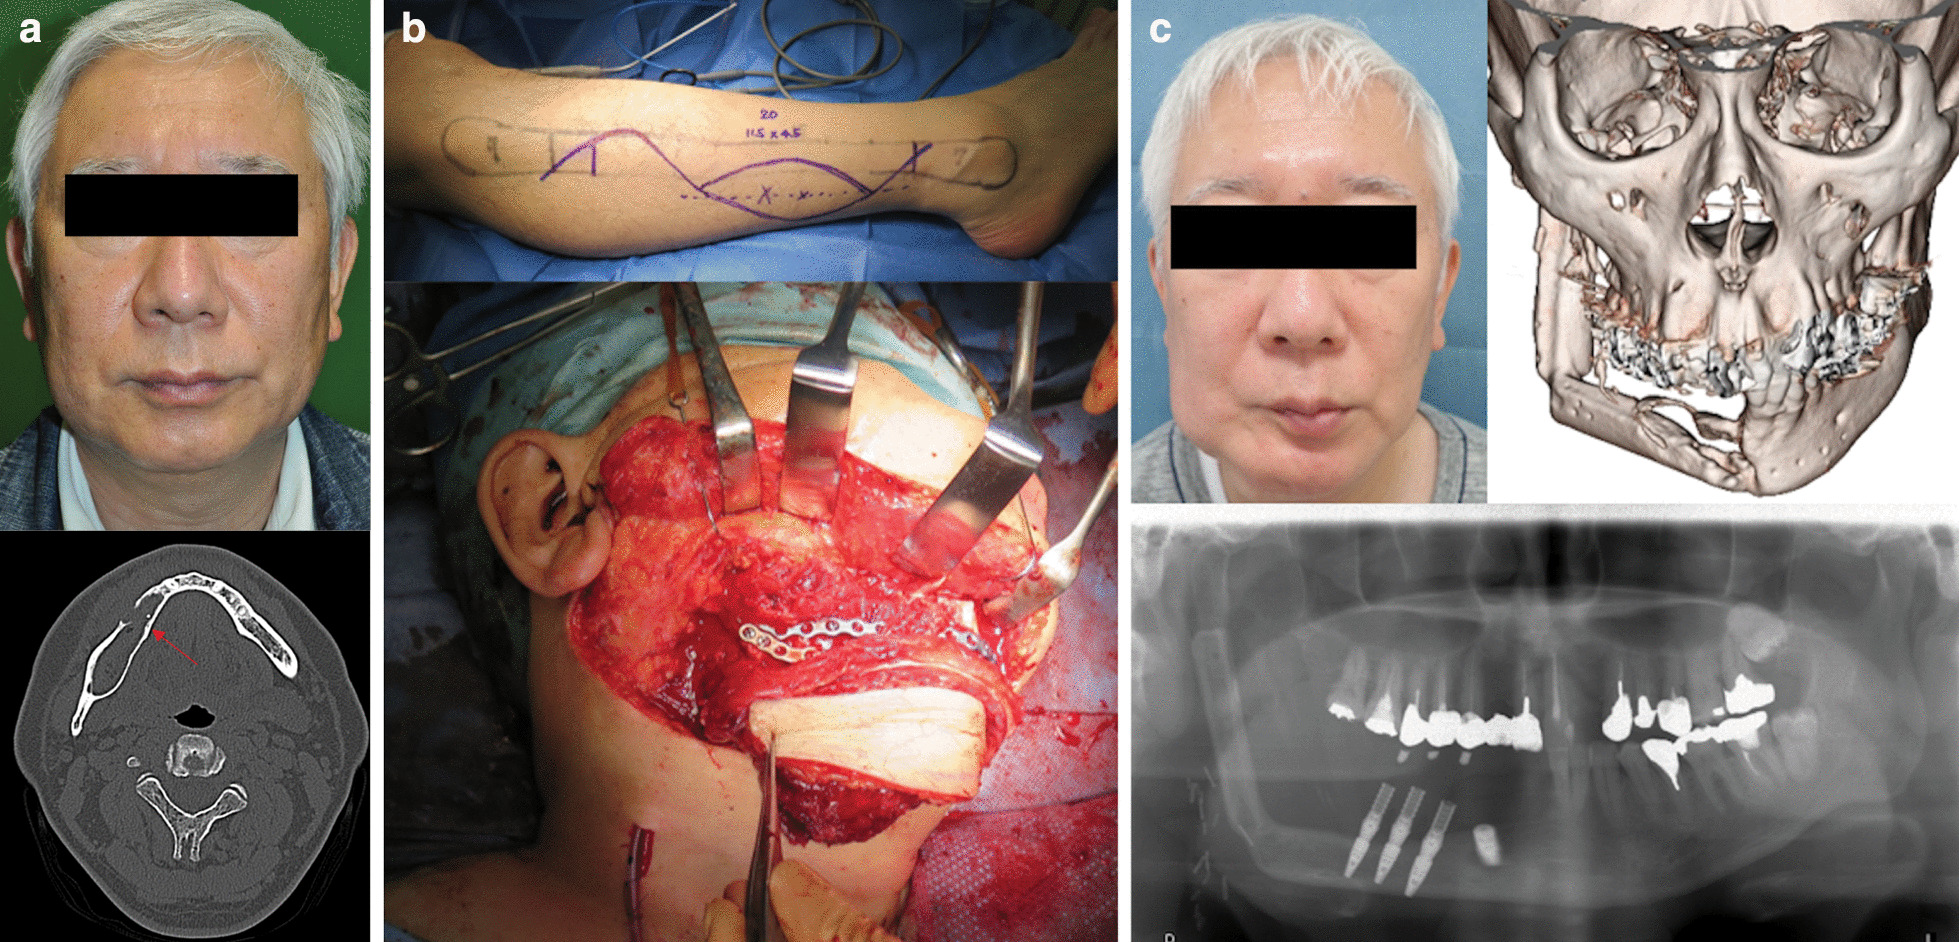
a Preoperative image of the patient 2 (right, patient 4 in Table 1). In the preoperative CT, an arrow shows the affected mandible with a calcifying odontogenic cyst (right). b Free osteo-cutaneous fibula flap harvested from the right lower limb. Osteotomy was performed in two places in the 18 cm long fibula (top). The harvested free osteo-cutaneous fibula flap was transplanted into the mandibular defect using the double barrel method (bottom). c Eleven months after surgery, although the distal part of the osteo flap developed osteonecrosis, there is no tumor recurrence and both functional and esthetical results were fair. This was achieved with a successful implant installation
